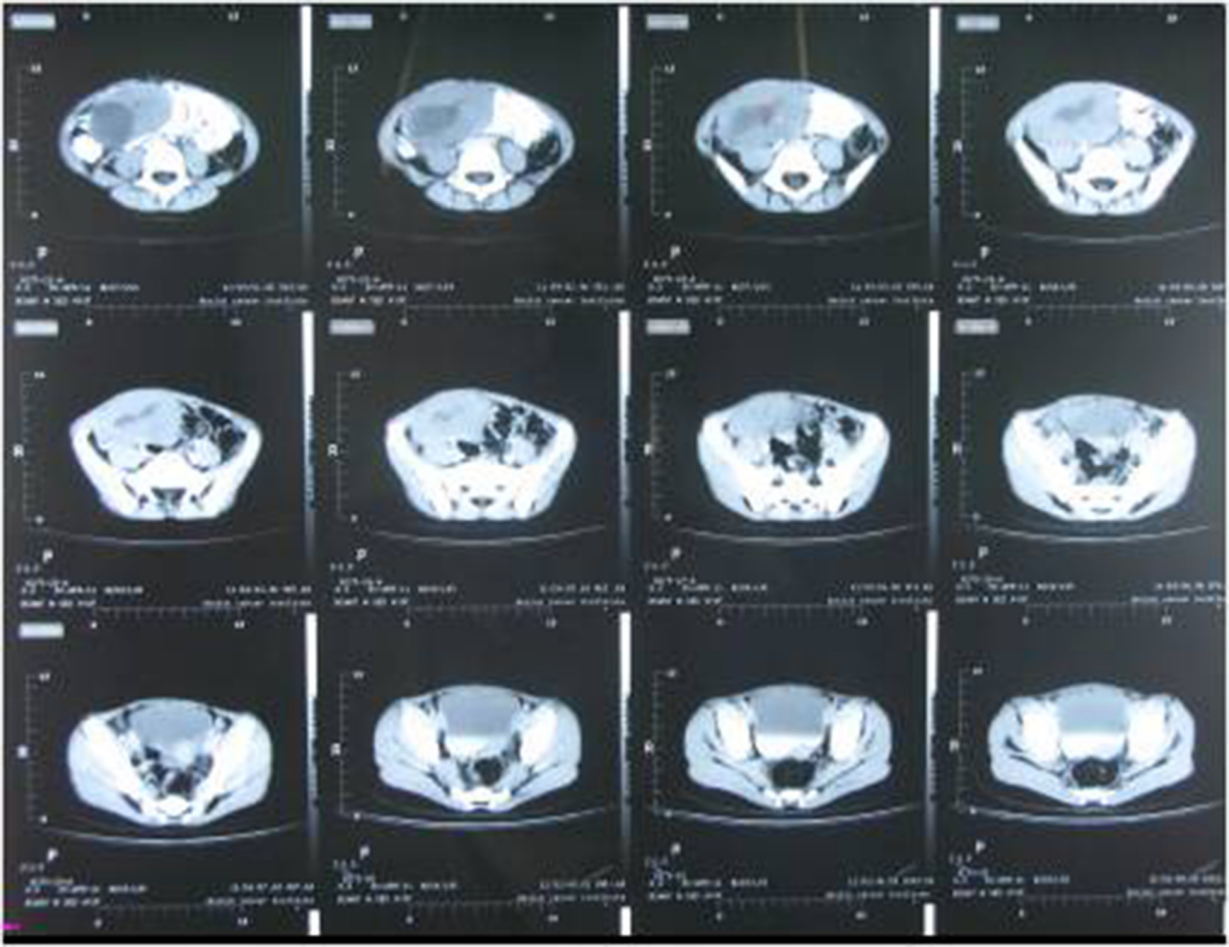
Computed tomography of the abdomen and pelvis shows a huge right ovarian mass.
